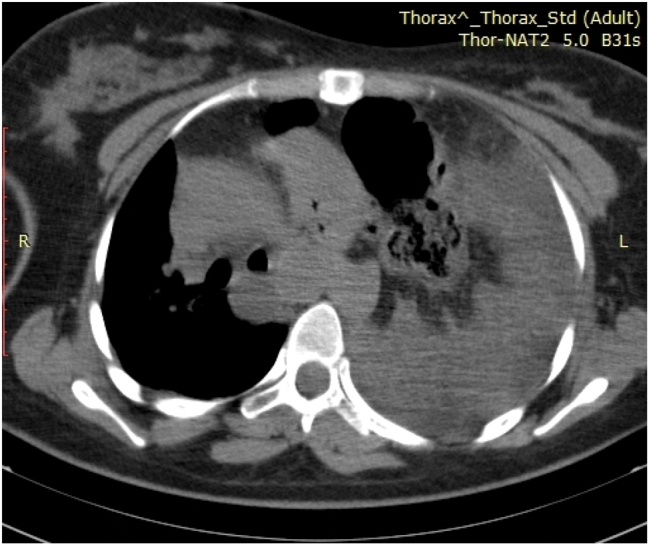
Image of a chest CT scan.
